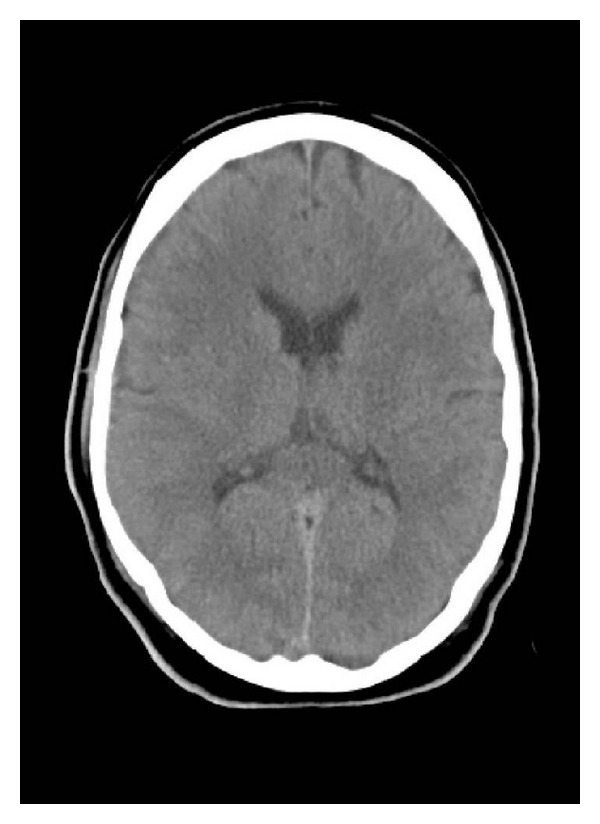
CT of the head without contrast reveals evidence of mild cerebral edema.
